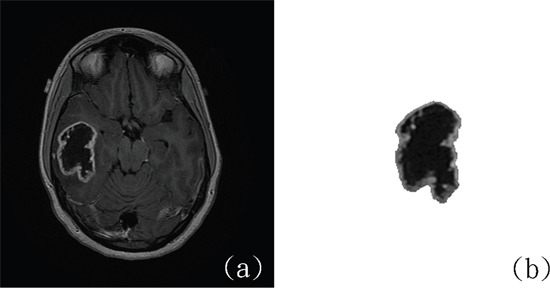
The only *IDH*-mutant case of GBM misclassified using the CAD system (a) Original MR image. (b) Delineated tumor area. (http://cancerimagingarchive.net/; “License” and the CC BY license, https://creativecommons.org/licenses/by/3.0/; tumor areas in this figure were extracted from original images.)
